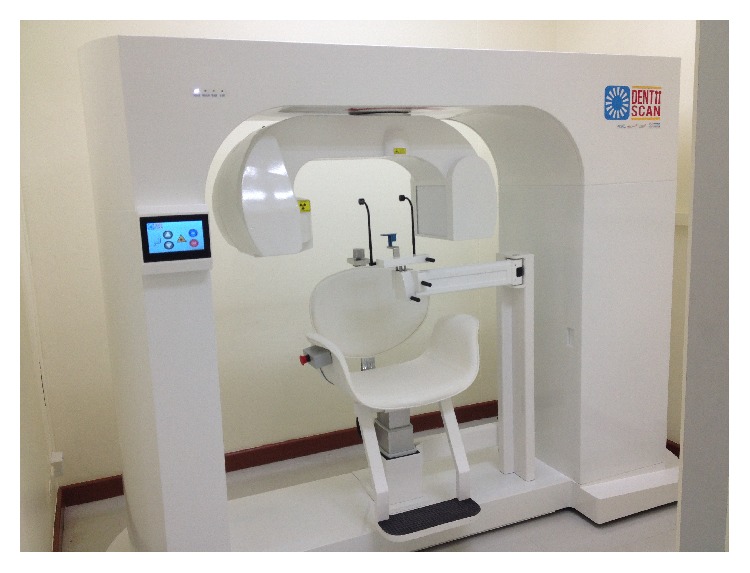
The dental CBCT scanner named DentiiScan 1.1.
